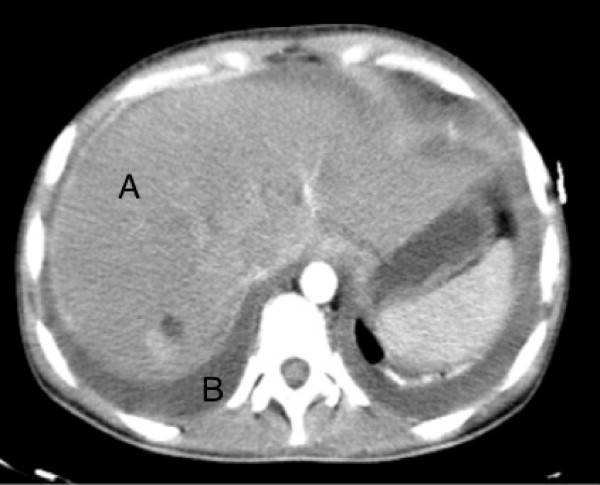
An enlarged liver and ascites. CT scan showing an enlarged and homogeneous liver (A) and ascites (B).
